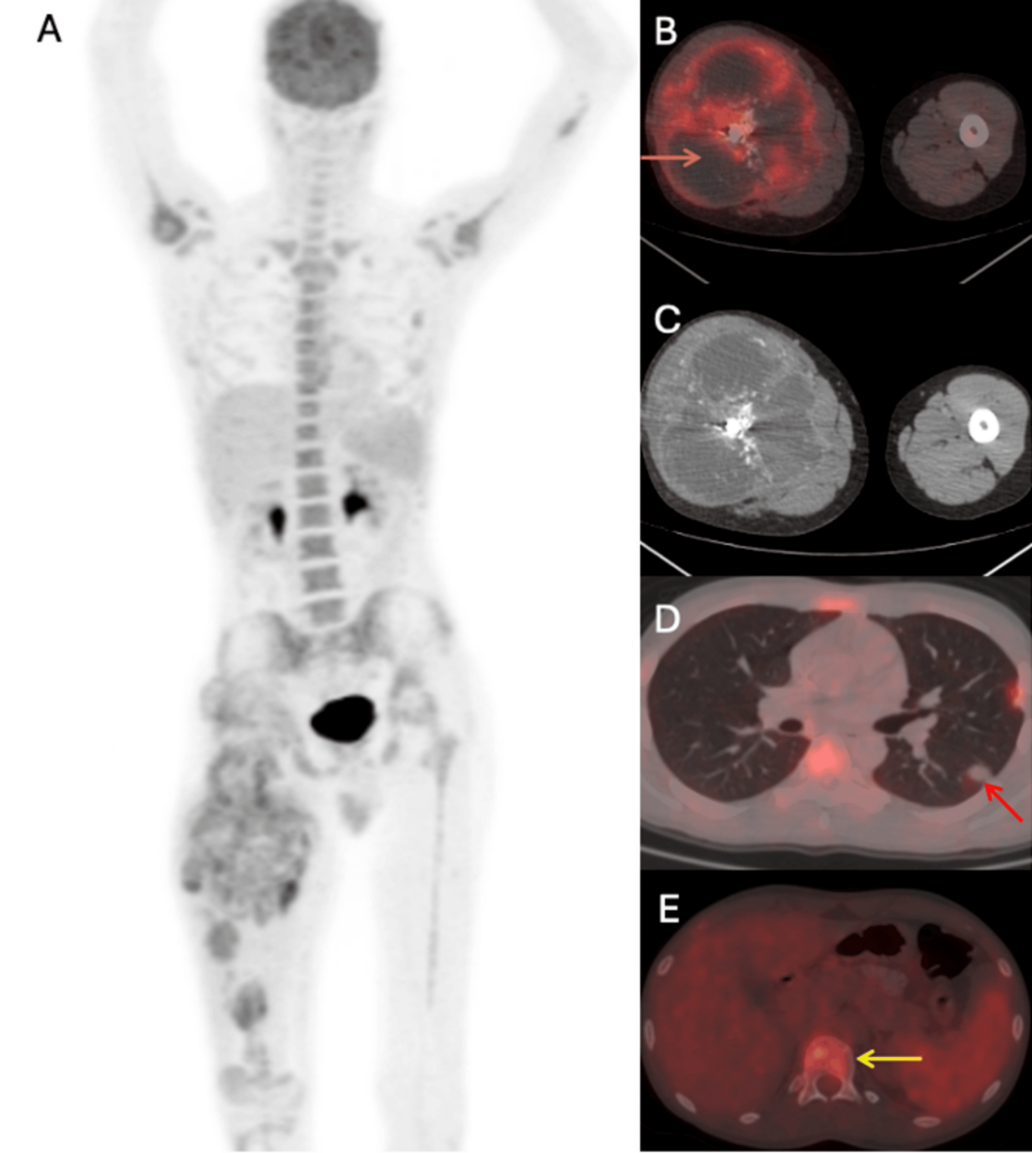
F-18 FDG PET/CT images F-18 fluorodeoxyglucose (FDG) Positron Emission Tomography (PET)/Computed Tomography (CT) images (maximum intensity projection (MIP) –  A, fused axial PET/CT – B, D & E, axial CT – C) for disease status; post intramedullary (IM) fixation of right femur, five cycles of chemotherapy and 10 fractions of radiotherapy, showing heterogeneously enhancing  soft tissue density mass with areas of necrosis and calcifications involving right gluteal and right thigh muscles (orange arrow), faintly FDG-avid peri-fissural left lung lower lobe nodule (red arrow) and multiple FDG-avid lytic-sclerotic lesions involving the skeleton (yellow arrow).
